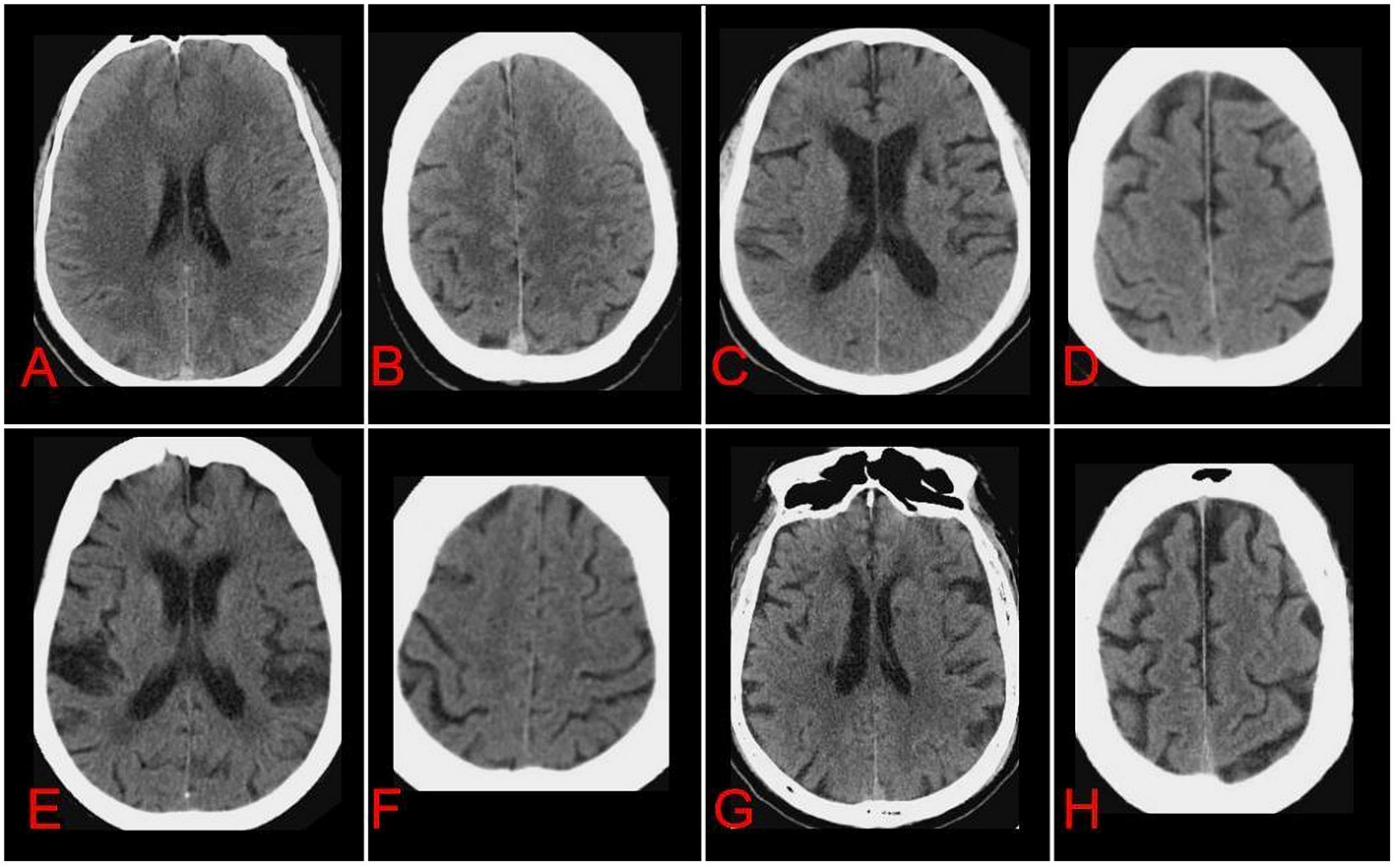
Axial non-contrast-enhanced CT images of brains from patients after acute ischemic stroke at the level of choroid plexus (A, C, E, G) or semioval center (B, D, F, H) of the lateral ventricle. Cerebral small-vessel disease (CSVD) markers were individually assessed and graded according to the appropriate scales, and total CSVD burden was calculated. Images in (A, B) are representative of patients with total CSVD burden of 0: anterior leukoaraiosis = 0, posterior leukoaraiosis = 0, no lacune, central atrophy = 0, cortical atrophy = 0. Images in (C, D) are representative of patients with total CSVD burden of 1: anterior leukoaraiosis = 1, posterior leukoaraiosis = 1, no lacune, central atrophy = 2, cortical atrophy = 1. Images in (E, F) are representative of patients with total CSVD burden of 2: anterior leukoaraiosis = 2, posterior leukoaraiosis = 2, no lacune, central atrophy = 2, cortical atrophy = 1. Images in (G, H) are representative of patients with total CSVD burden of 3: anterior leukoaraiosis = 2, posterior leukoaraiosis = 1, lacune in left and right basal ganglia = 2, central atrophy = 2, cortical atrophy = 2.
